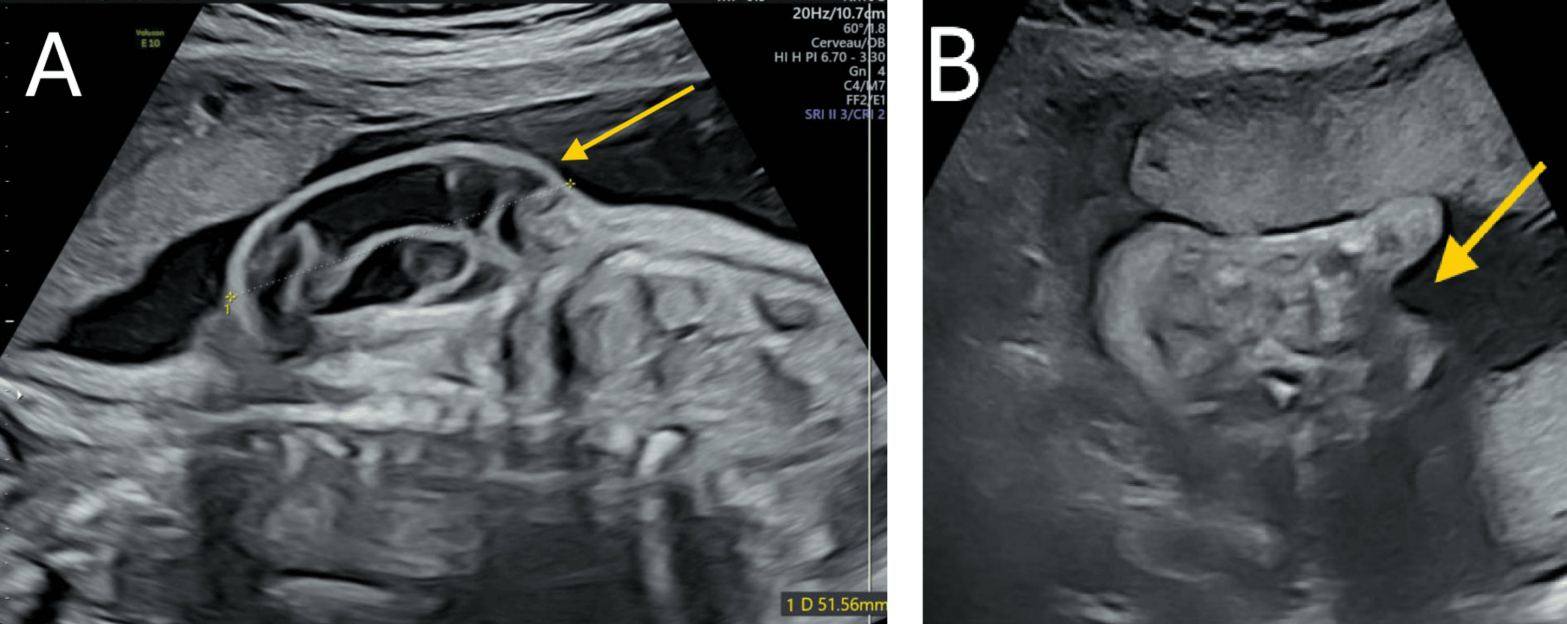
Fetal ultrasound images at 22 weeks and six days of gestation A: posterior subcutaneous fluid-filled mass (arrow) along the thoracic spine; B: sandal gap (arrow)
